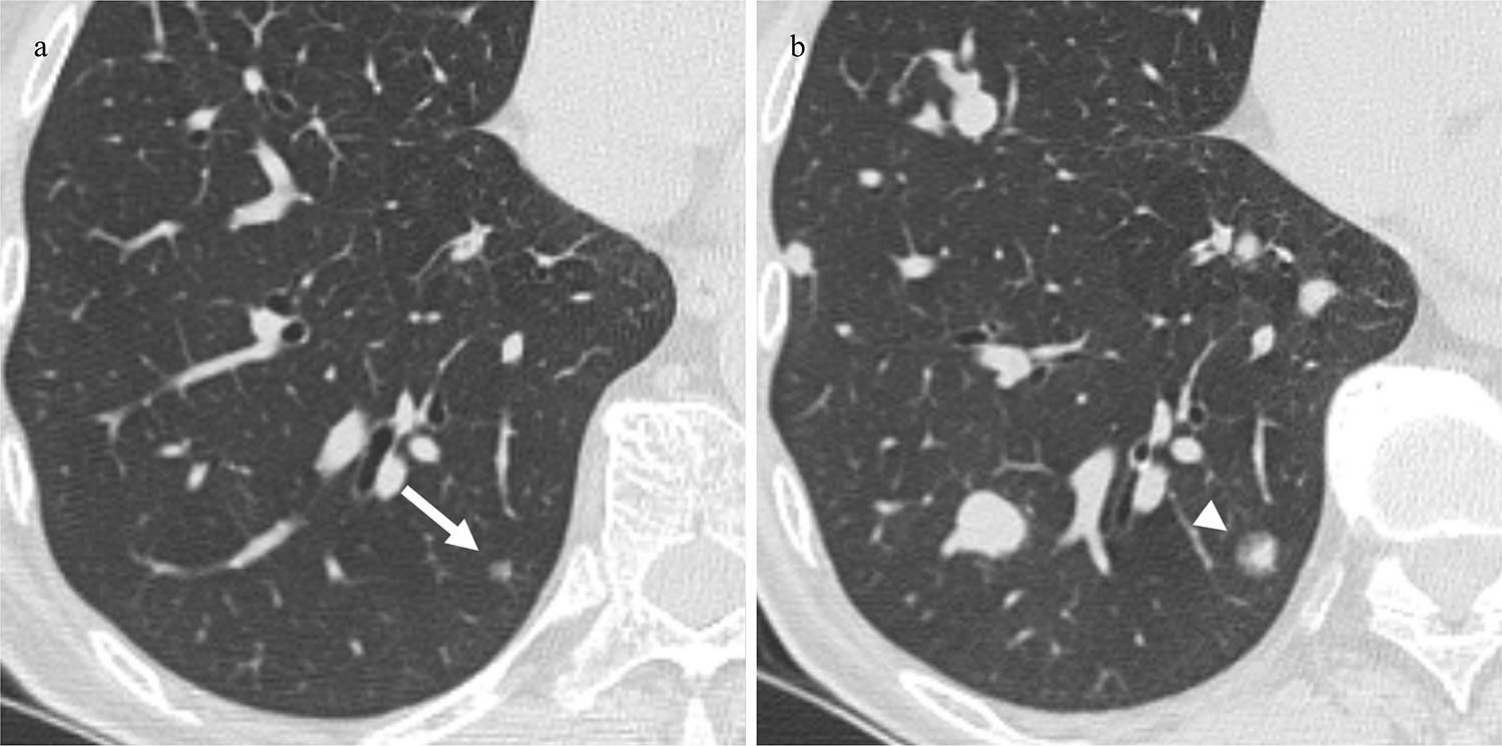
A 67-year-old woman with mucosal melanoma. a. CT 24 months after the operation shows 3 mm-sized pure GGN in the right lower lobe (white arrow). b. After 6 months, the GGN had grown to 8 mm and turned into a part-solid nodule (white arrowhead). This nodule resembled a lung adenocarcinoma; however, it grew at a faster rate than a lung adenocarcinoma. In addition to this nodule, there were multiple fast-growing GGNs and solid nodules in bilateral lungs (not shown). This nodule was diagnosed as lung metastasis
